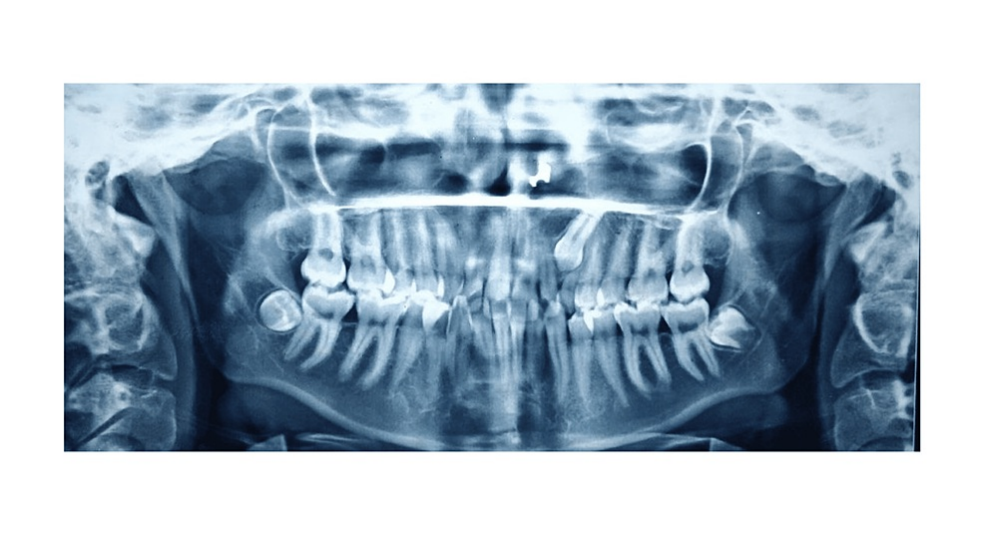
Pre-treatment OPG (orthopantomogram)
